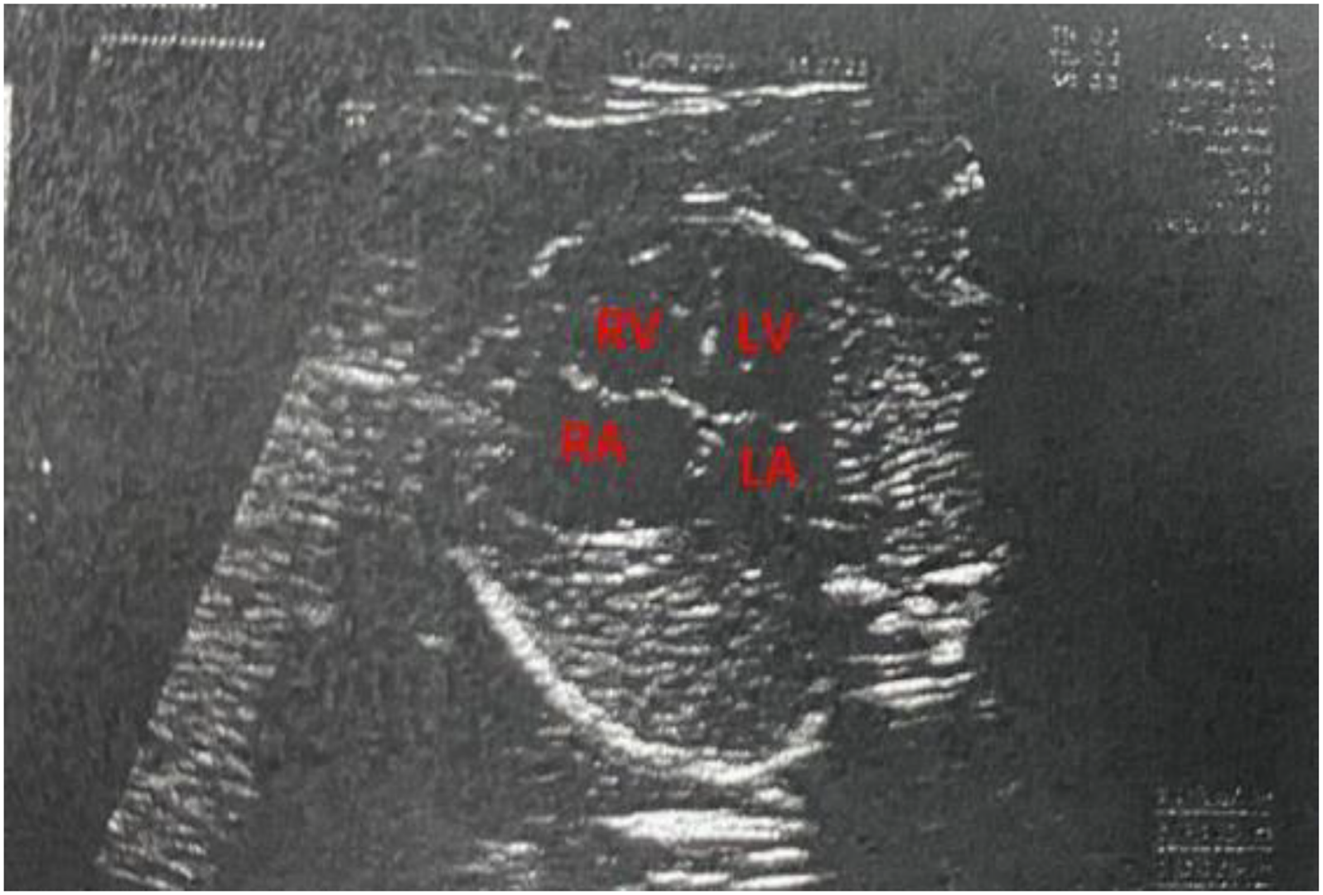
A fetal echocardiogram at 25 weeks of pregnancy. RV, right ventricle; RA, right atrium; LV, left ventricle; LA, left atrium.
